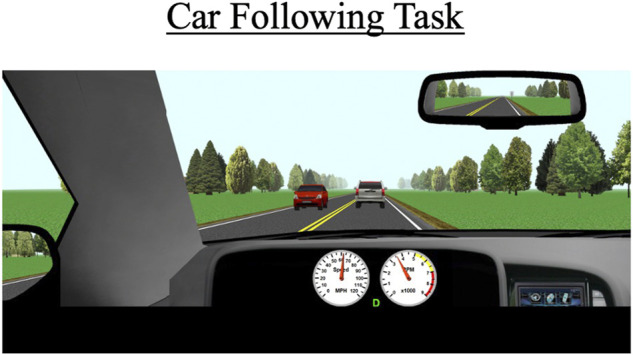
The car-following task occurs behind a white SUV over a duration of 5 min. The primary outcomes for this scenario are standard deviation of lateral position (SDLP), the car’s modulus, and the coherence to the task.
